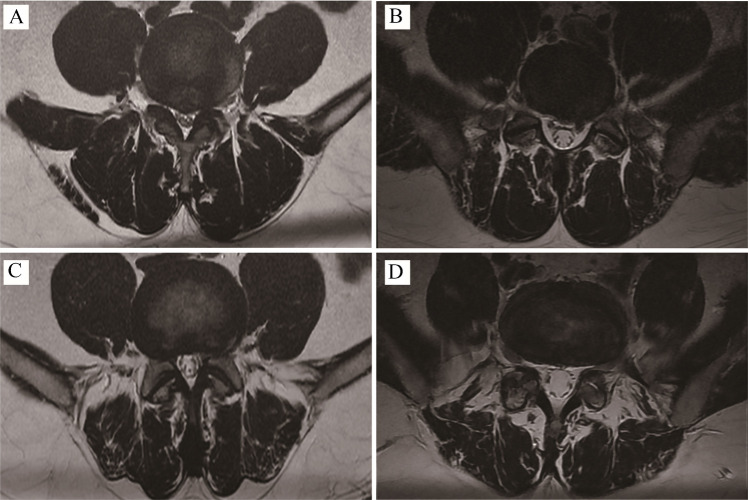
MRI classification of disc herniation. (A) Central type; (B) Lateral recess type; (C) Foraminal type; (D) Extreme lateral type
